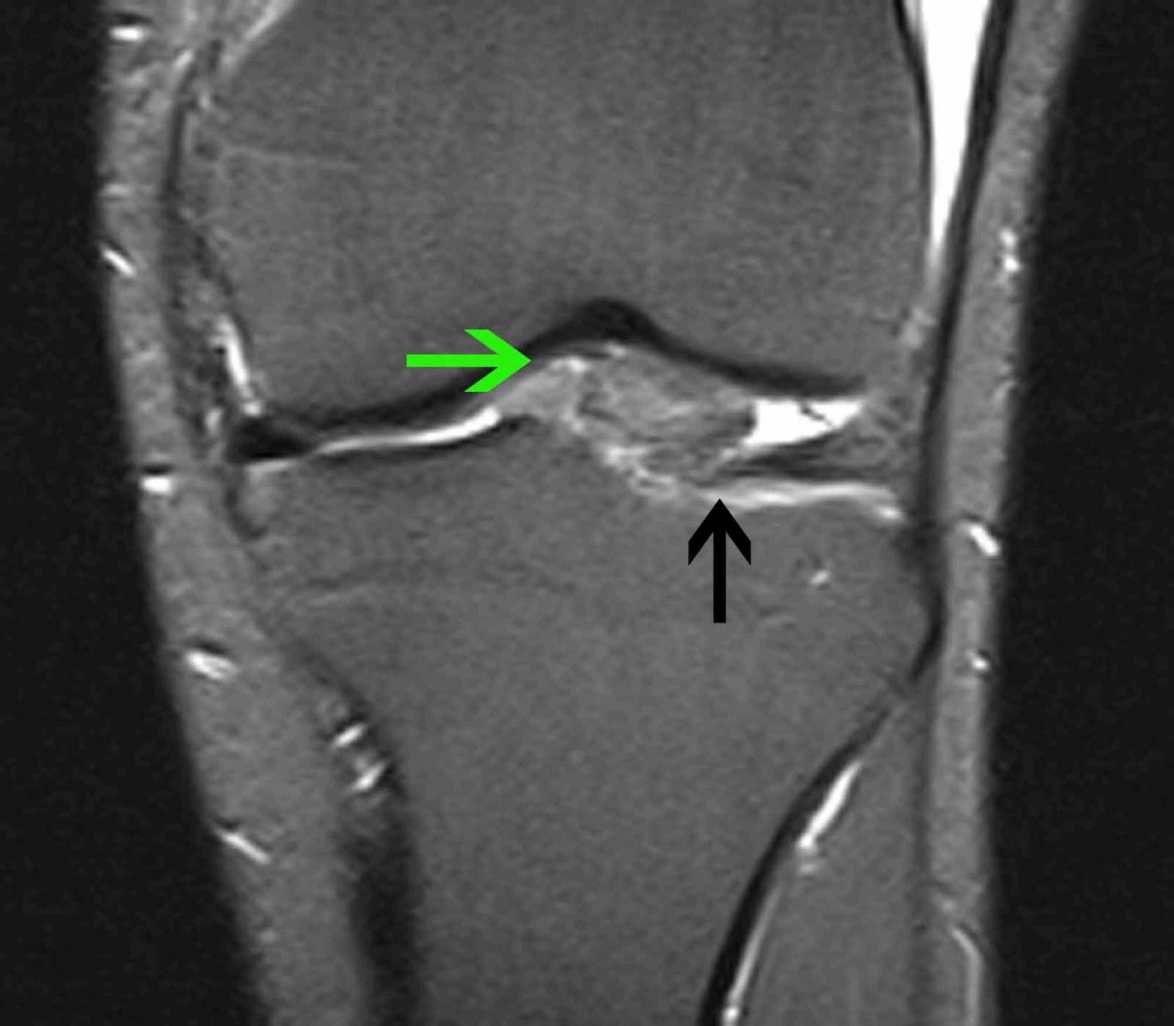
MRI of the left knee (coronal plane) T1-weighted turbo inversion recovery magnitude (TIRM) sequence, coronal plane, in which the mass can be seen between the lateral femoral condyle and the lateral tibial plateau (green arrow). It appears to be arising from the anterior attachment of the lateral meniscus (black arrow).
